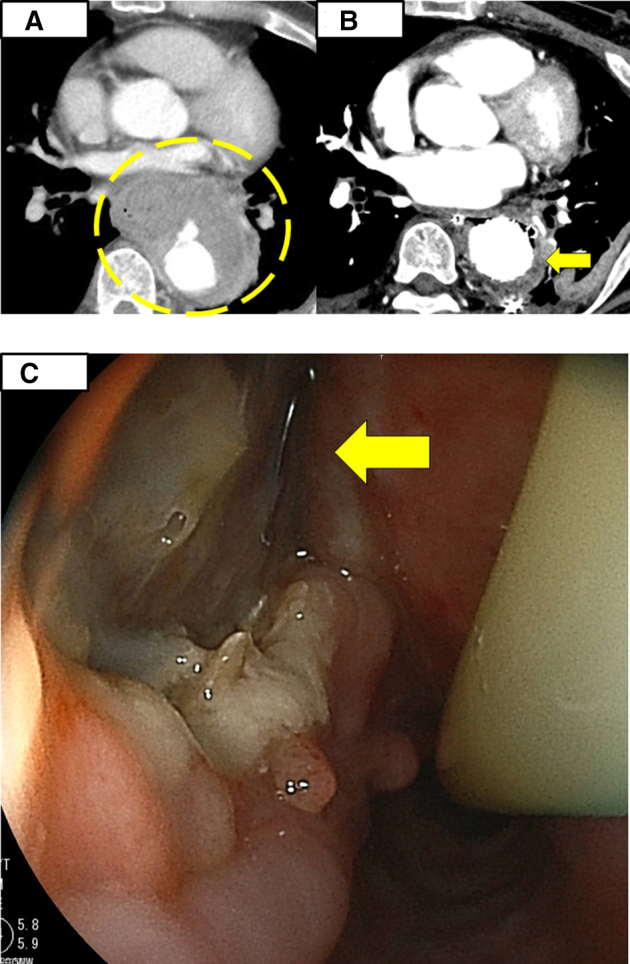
A, Computed tomography (CT) image showing a ruptured descending aorta (*yellow circle*). B, CT image showing free air surrounding the stent graft in the descending aorta (*yellow arrow*). C, Endoscopic image of the mid-esophagus revealing a fistula with the visible stent graft (*yellow arrow*).
